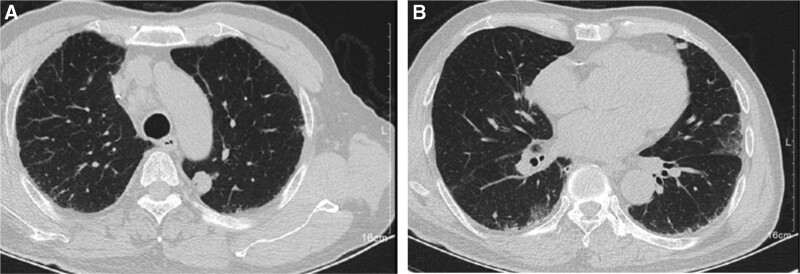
Chest computed tomography (CT) on November 28, 2020. Some ground-glass changes occurred in the lungs.
